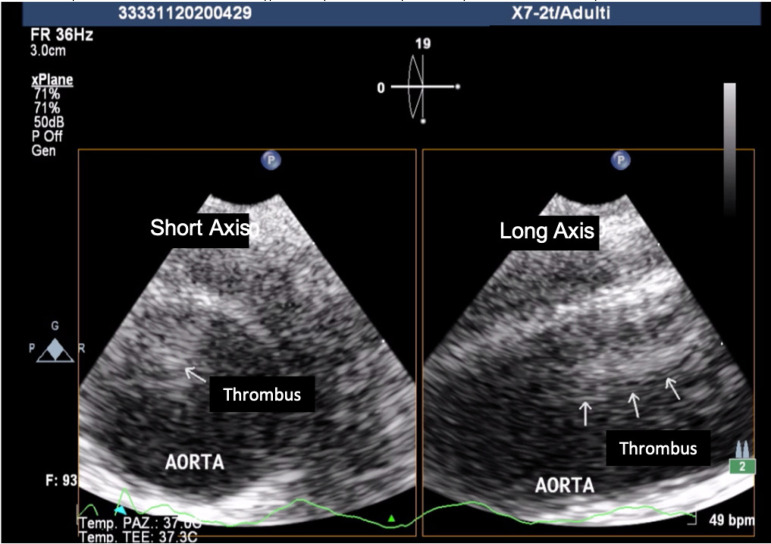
Follow-up transesophageal echocardiography showing no modifications of aortic mural thrombus in short axis (left) and long axis (right).
